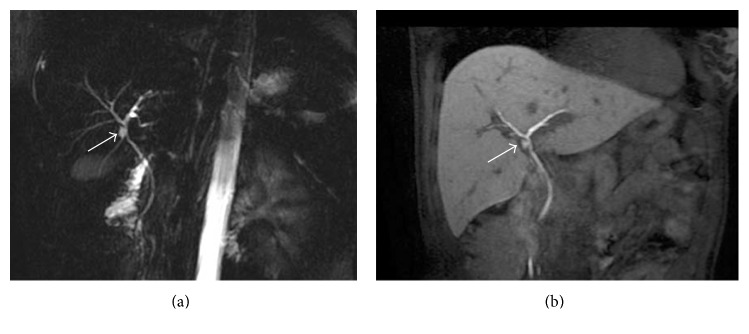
Biliary cystic disease, type II. MRCP image (a) shows a small paracholedochal cyst, with homogeneous hyperintense signal (white arrow). 3D T1-weighted spoiled gradient echo image (b)—obtained in an excretory phase—shows opacification of the cystic lesion (white arrow); a diagnosis of type II biliary cystic disease was achieved.
